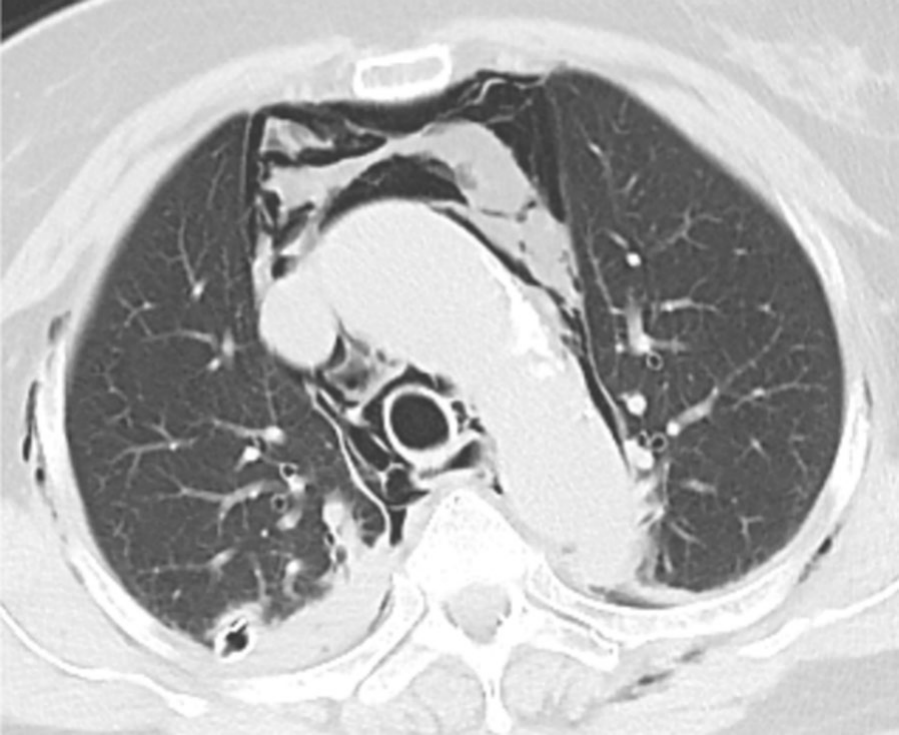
Postoperative CT revealed mediastinal and subcutaneous emphysema without any evident tracheal injury
